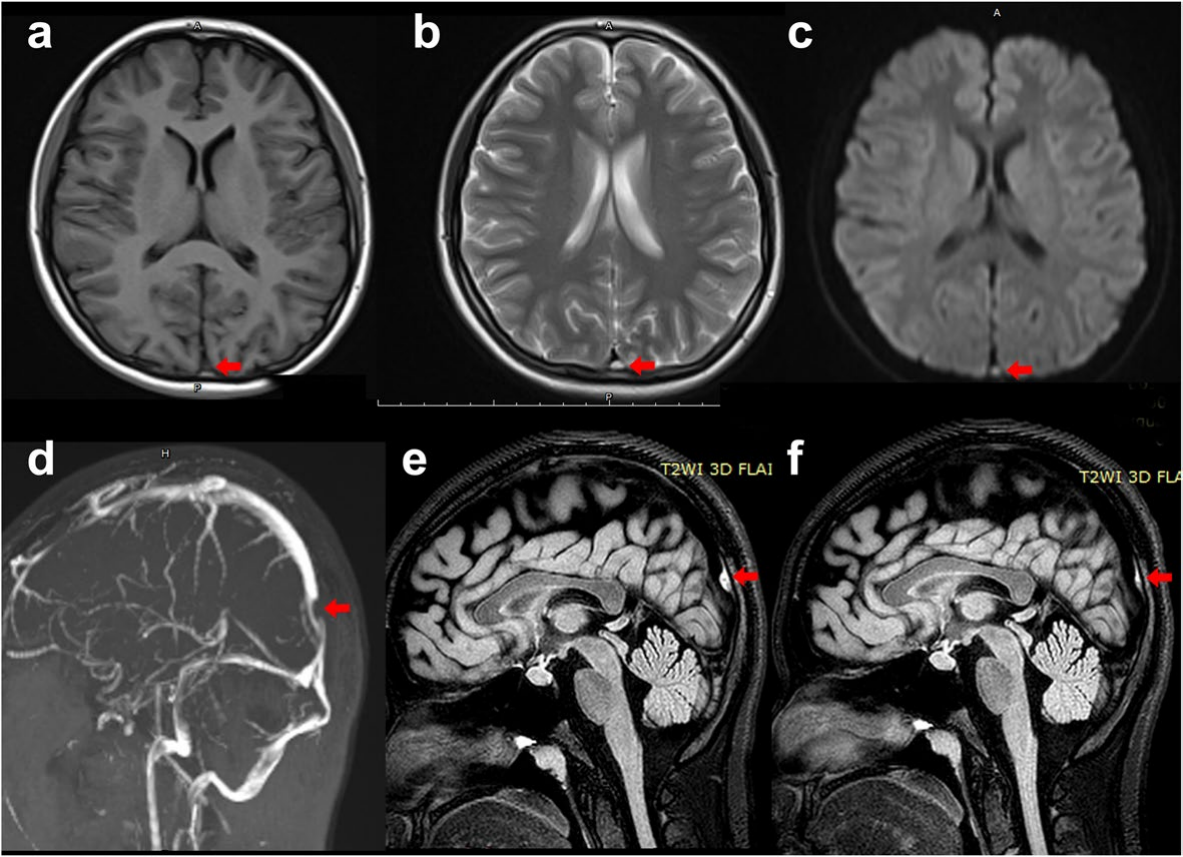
Cerebral MRI and MRV of the patient Magnetic resonance imaging (MRI) showed abnormal signal lesions in the superior sagittal sinus, which was isointense on TW1I (a) and hyperintense on TW2I (b) and diffusion-weighted images (c). Magnetic resonance venography (MRV) strongly suggested stenosis of superior sagittal sinus (d) MRI showed stenosis of superior sagittal sinus and abnormal signal lesions in the superior sagittal sinus, which was hyperintense in fluid attenuated inversion recovery (FLAIR) after admission (e). After one month, MRI showed shrinkage of the thrombosis in the superior sagittal sinus; the degree of stenosis had significantly improved (f)
